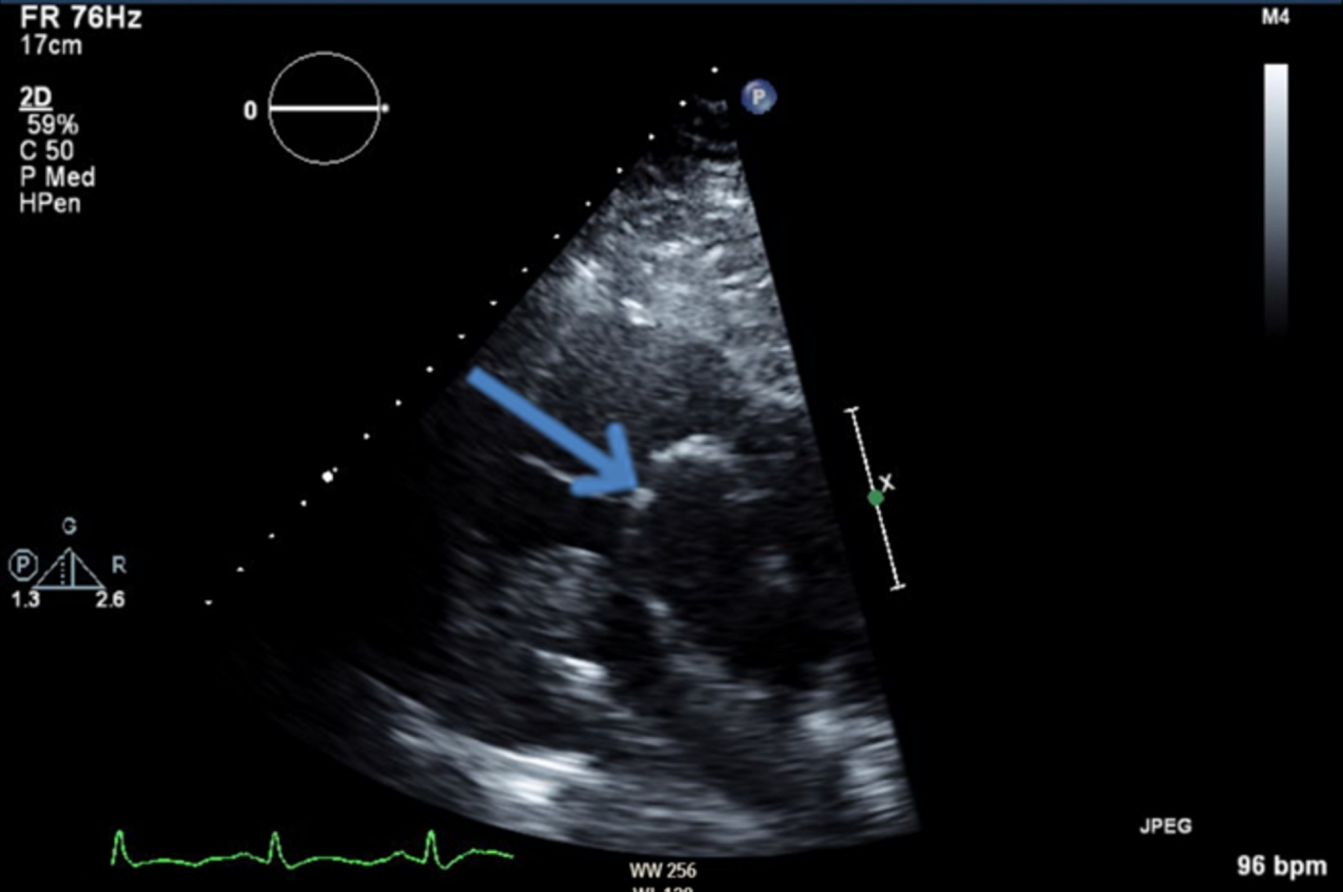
Echocardiography - short axis view at the aortic valve level showing the right atrial mass
